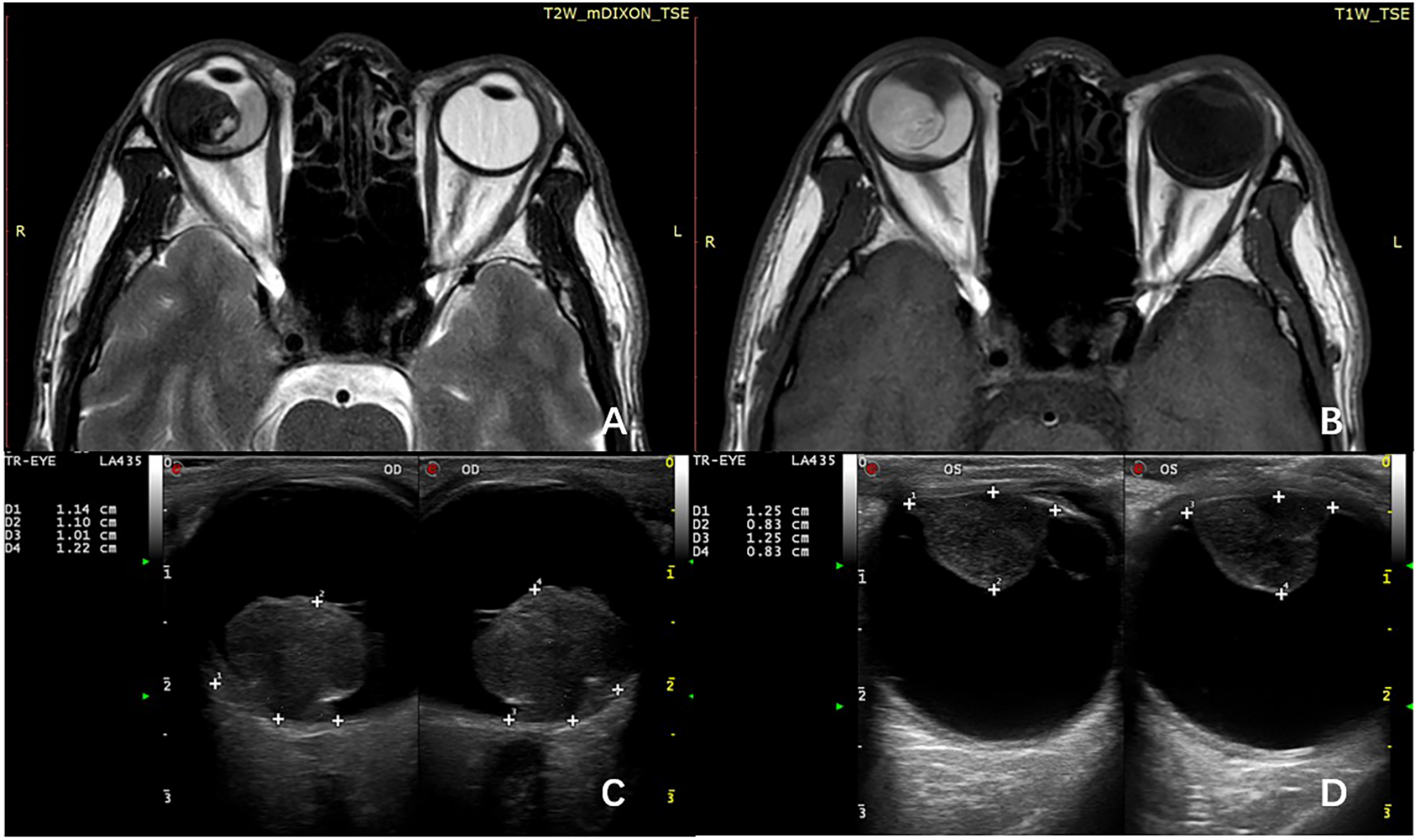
MRI shows the primary tumor has spread to the ciliary body on T1-weighted image (A) and T2-weighted image (B). B-scan ultrasonography can roundly measure the size of choroid melanoma (C) and ciliary body melanoma (D).
